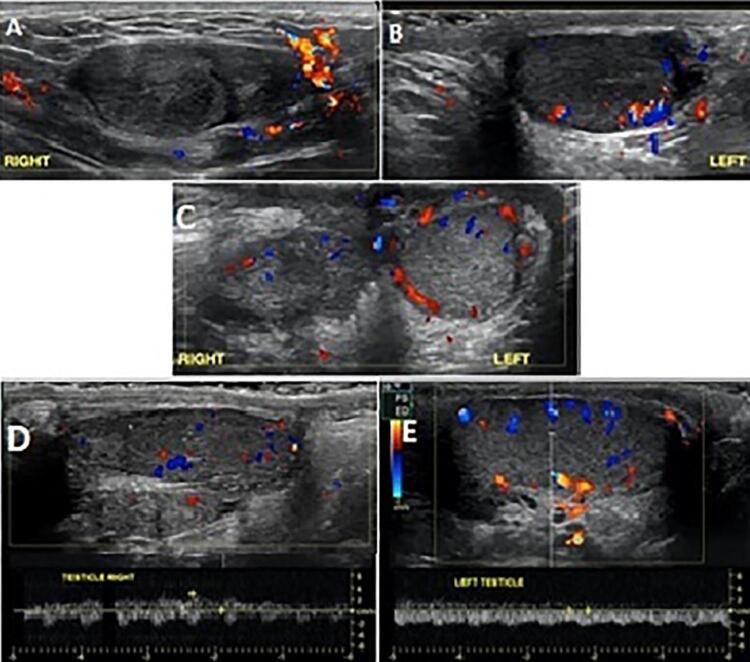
Pre and post testicular ultrasounds in a 14-year old boy with borderline viable testis after detorsion who underwent right orchio-septopexy.
